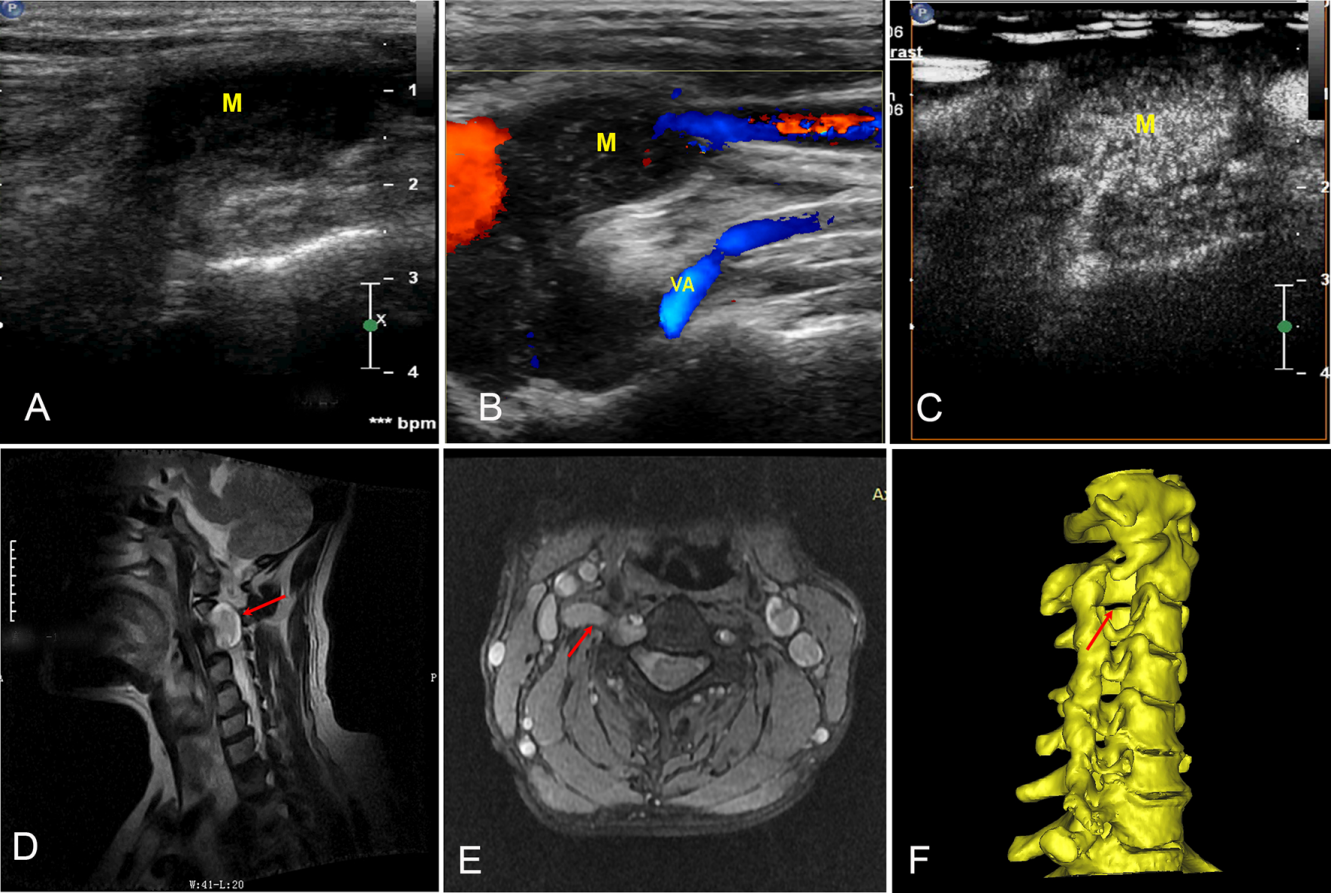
MRI, CT and contrast ultrasound images of cervical plexus schwannomas (C3 level). A 2D ultrasonography of the transverse process of the vertebra, where M is the neuroma lesion originating from the intervertebral foramen. B Colour Doppler ultrasonography showing the blood flow signal in the mass. C Contrast-enhanced ultrasound showing rich blood perfusion. D, E MRI sagittal and cross-sections of a CP mass: the arrow indicates the lesion growing outwards from the intervertebral foramen. F The arrow indicates the enlarged intervertebral foramen (3D CT reconstruction)
